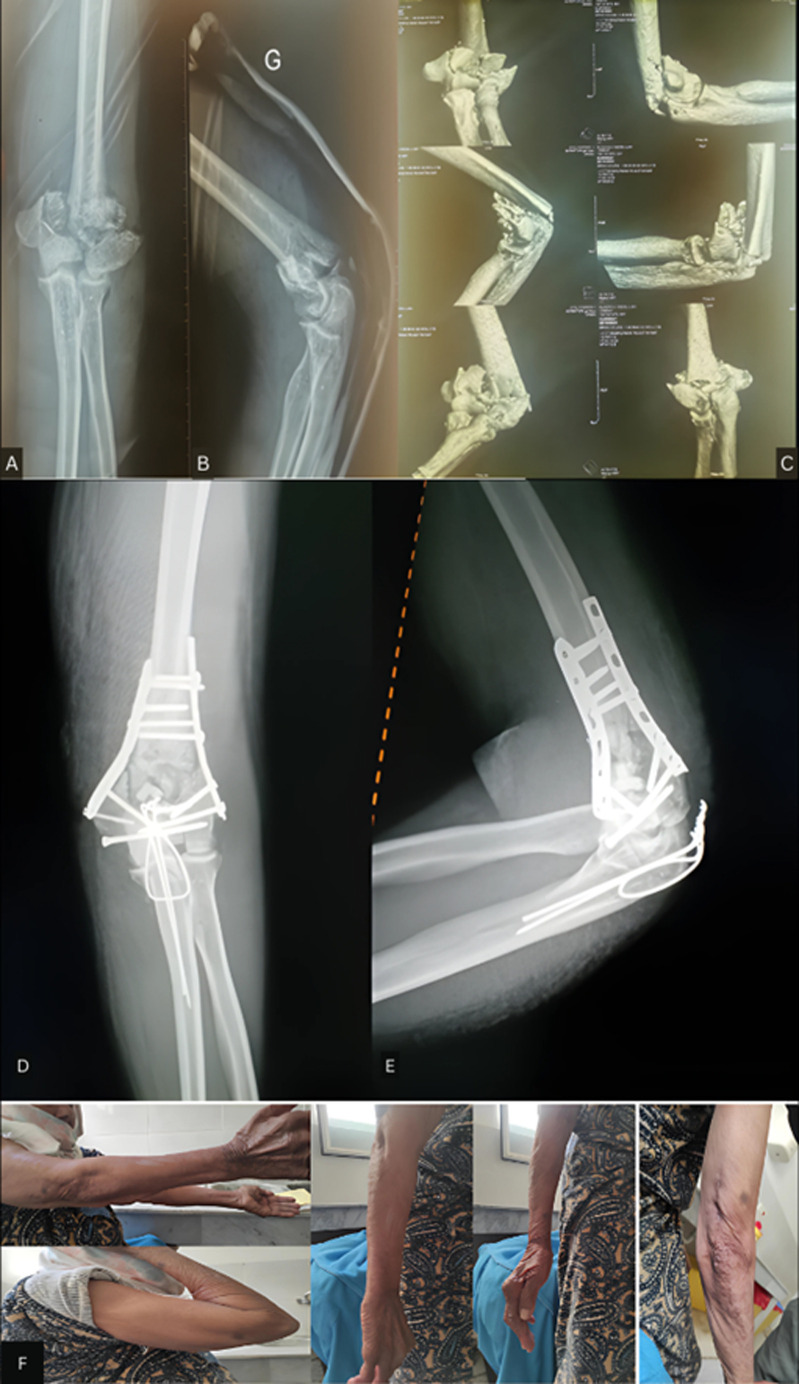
complex articular fracture; A,B) classification type C, standard elbow radiograph, anteroposterior and lateral views; C) 3D CT scan; D,E) dual plate parallel osteosynthesis with initial simplification by direct epiphyseal screwing and final bridging fixation of the olecranon osteotomy; F) highly satisfactory outcome (flexion, extension, and pronosupination) with good healing after 4 months of follow-up
